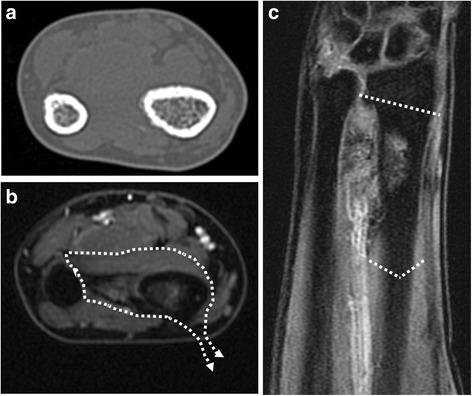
After neoadjuvant chemotherapy. a CT axial image (bone condition). Periosteal reaction was detected prior to chemotherapy. b, c Gadolinium contrast MRI; (b) axial and (c) coronal images. The soft tissue extension of the tumor shrunk remarkably and a small enhanced lesion was detected in the radius and intraosseous membrane. The dotted arrow indicates the resection range including the radius, intraosseous membrane, and pronator quadratus muscle. The dotted line indicates the osteotomy line of the radius
